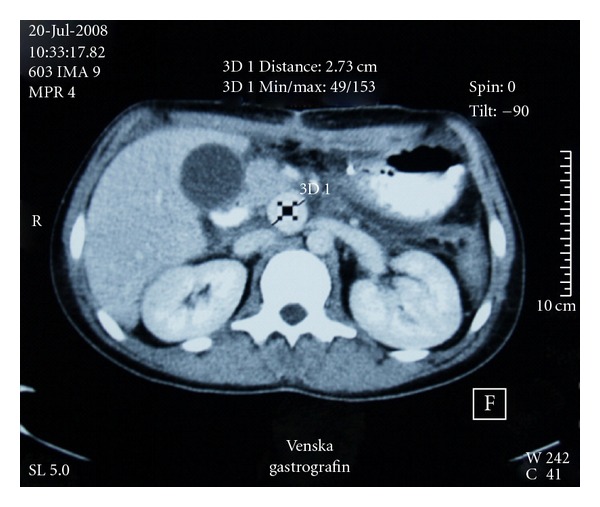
25-years-old male patient with SMA pseudoaneurysm. Transverse CT scan in delayed venous phase (45 seconds), slice thickness showing oval hyper vascular lesion between aorta and inferior vena cava 27 mm in diameter.
